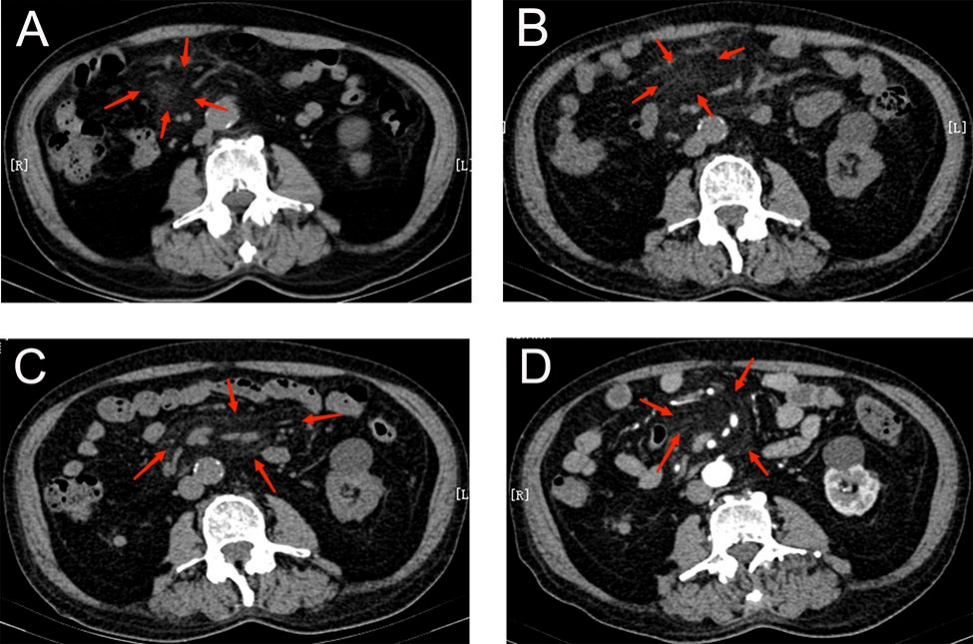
A 96-year-old man with abdominal pain on the right side. Plain abdominal CT revealed hyper-attenuation of mesenteric fat (A). After antibiotic treatment, the abdominal pain was mitigated but soon worsened (B). The abdominal pain was gradually controlled following the initiation of prednisone therapy (40 mg daily), repeat CT revealed less panniculitis (C), and contrast-enhanced CT [iodinated contrast agent, Iohexl Injection, GE HEALTHCARE (Shanghai) Co., Ltd., China] revealed hyper-attenuation of mesenteric root, accompanied by a fat halo sign that surrounded the vessels, which suggested MP (D)
